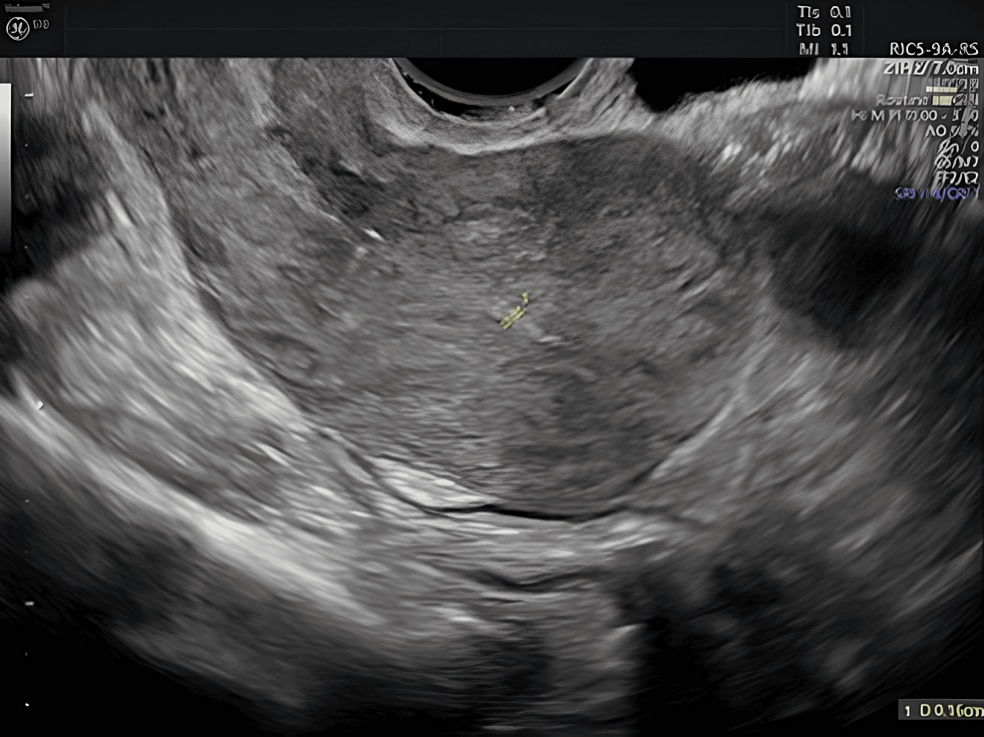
Transvaginal ultrasound sagittal scan of the uterus: thin linear homogeneous hyperechoic endometrium of 1.6 mm; regular homogeneous hypoechoic myometrium. Absence of endocavitary fluid.
